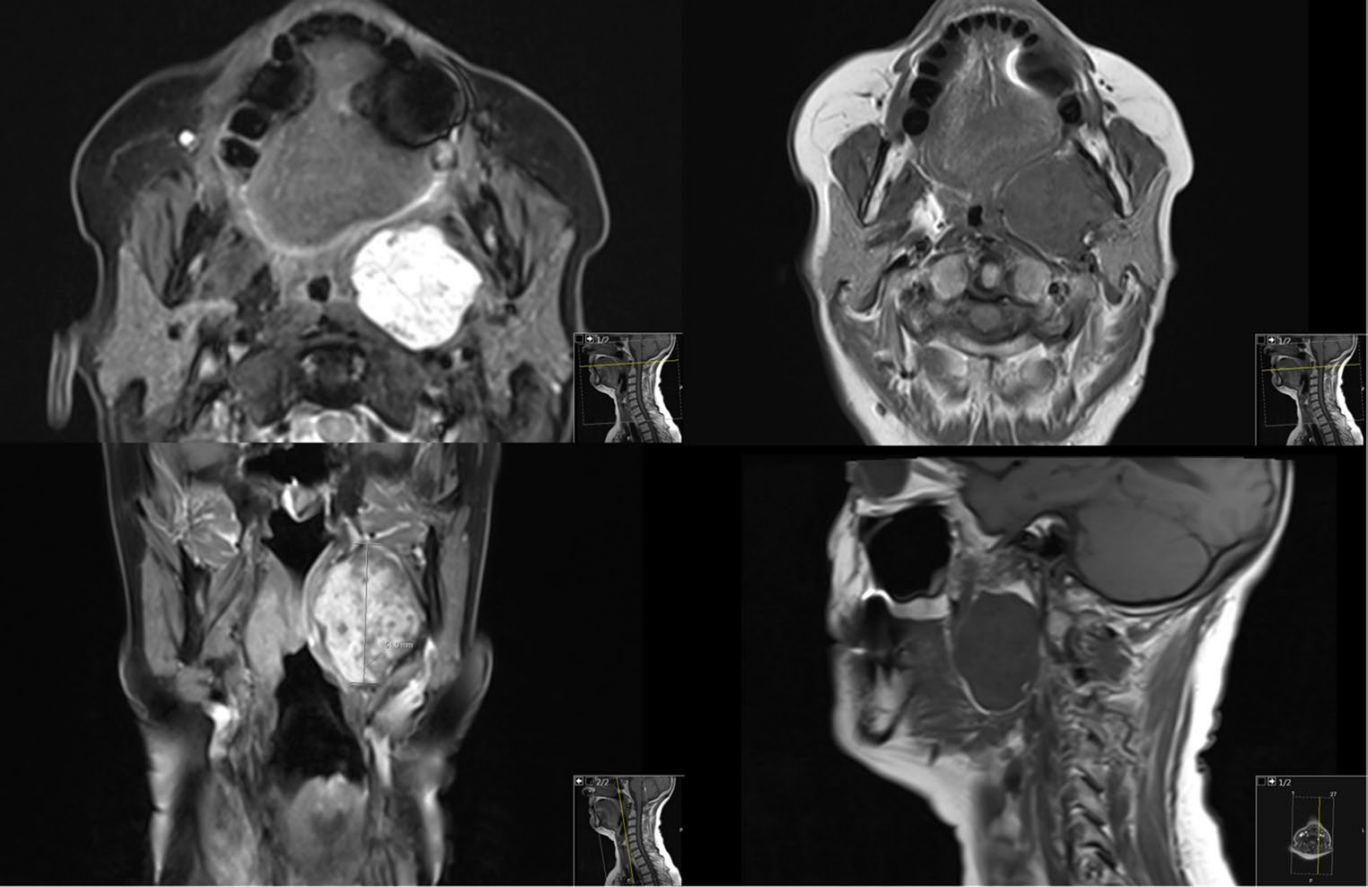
MRI Neck with contrast (axial, coronal and sagittal views with different weightings)
